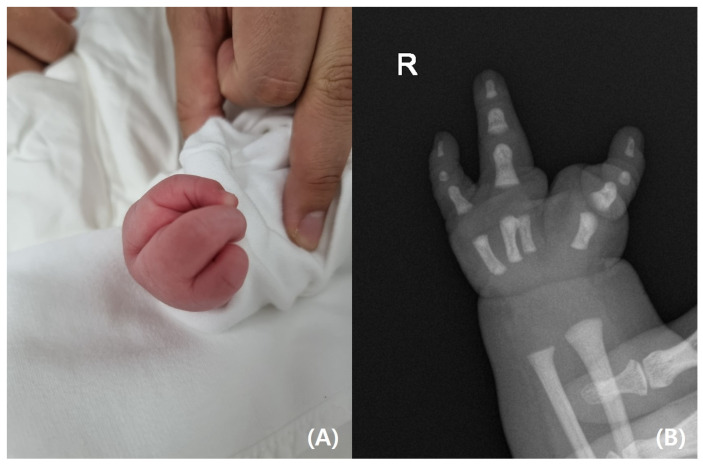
(A) Ectrodactyly of the right hand. (B) Radiograph of the right hand showing tetradactylous ectodactyly.
